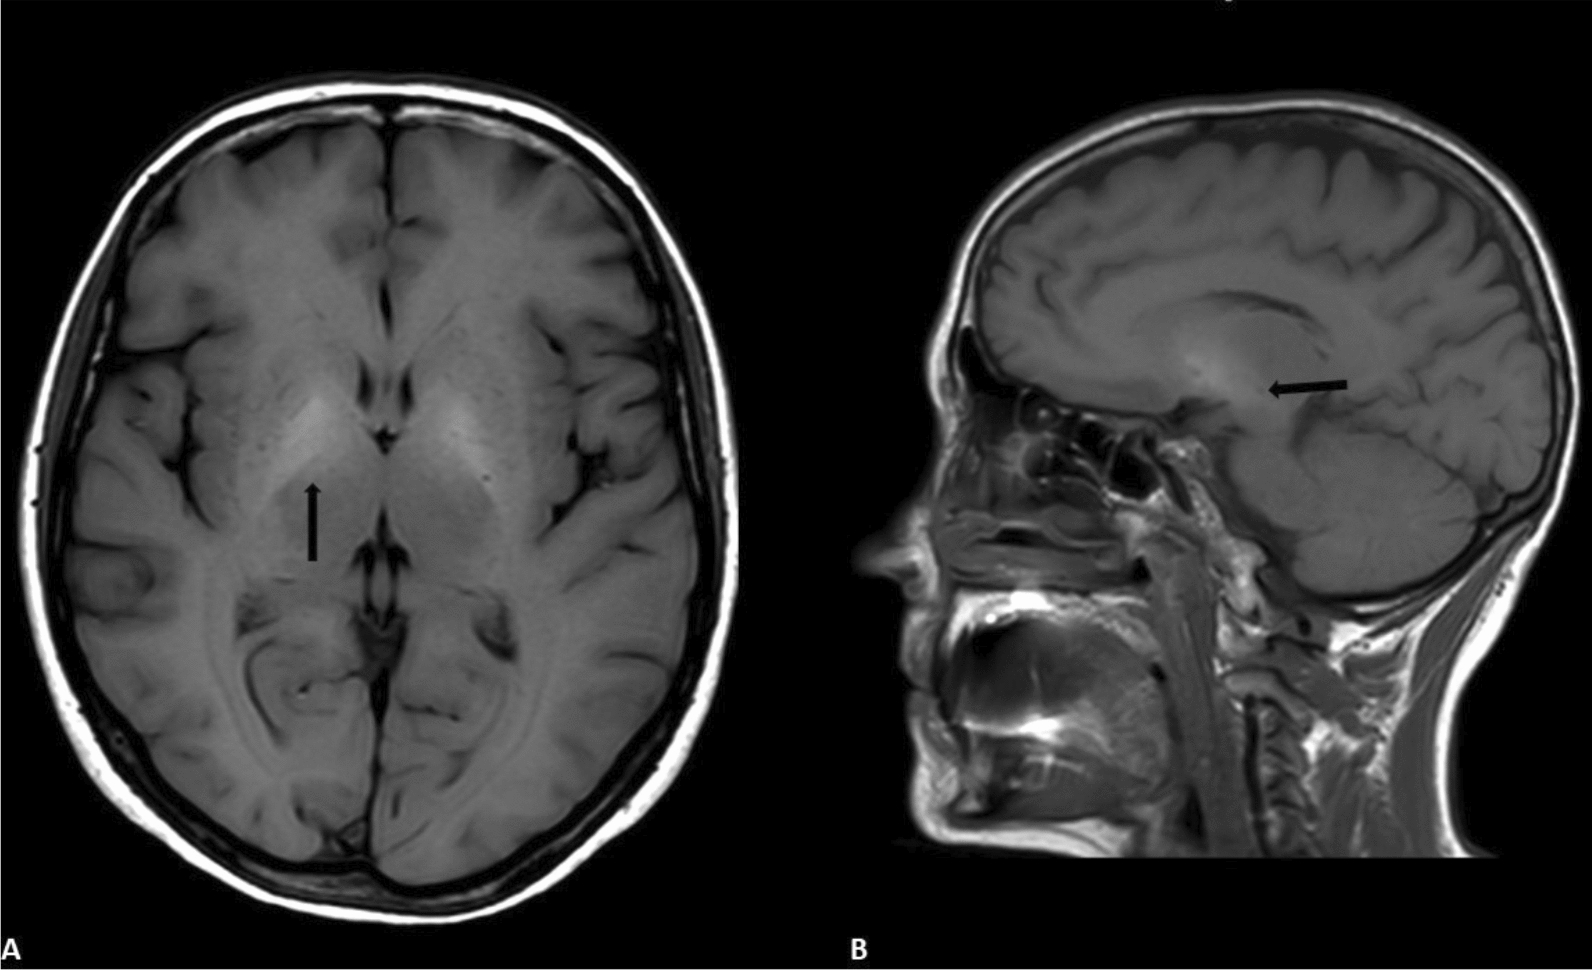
T1-weighted imaging showing high signal intensity in the basal ganglia without mass effect, which results from paramagnetic manganese deposition (A: axial plane; B: sagital plane)
